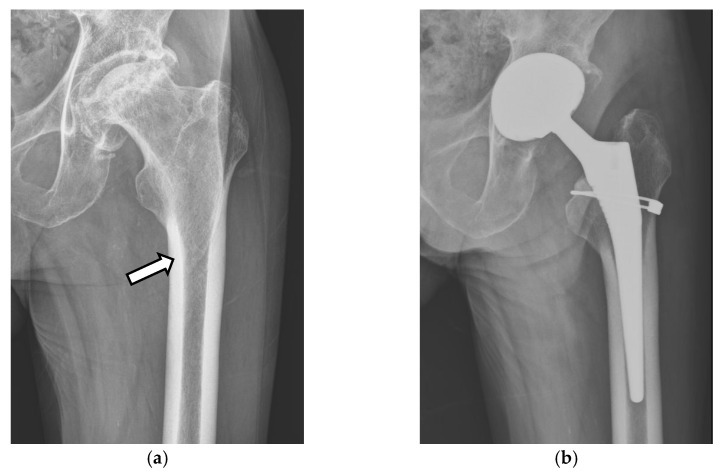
Preoperative and postoperative radiographs of a 78-year-old male with osteonecrosis of the femoral head treated with total hip arthroplasty using a type B2 quadrangular tapered stem. (a) Preoperative radiograph demonstrating Dorr type A femoral morphology with a narrow femoral canal and thick cortices (arrow). (b) Postoperative radiograph showing well-fixed stem with cerclage wiring.
